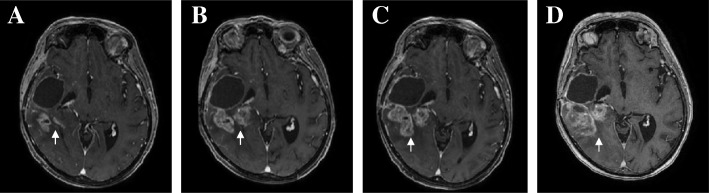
Magnetic resonance imaging. Gadolinium-contrast-enhanced T1-weighted 3D magnetic resonance imaging of the brain after radiotherapy (a), before afatinib treatment (b), and after 4 weeks (c) and 8 weeks (d) of afatinib treatment. The lesion is indicated by an arrow
